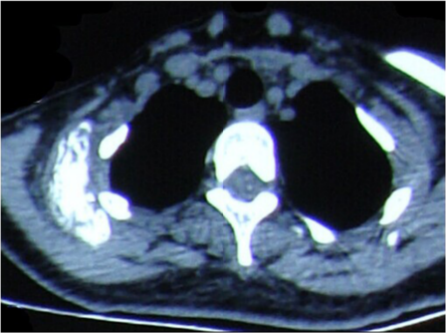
Axial computed tomography scan image showing a mixed density shadow in the serratus anterior
